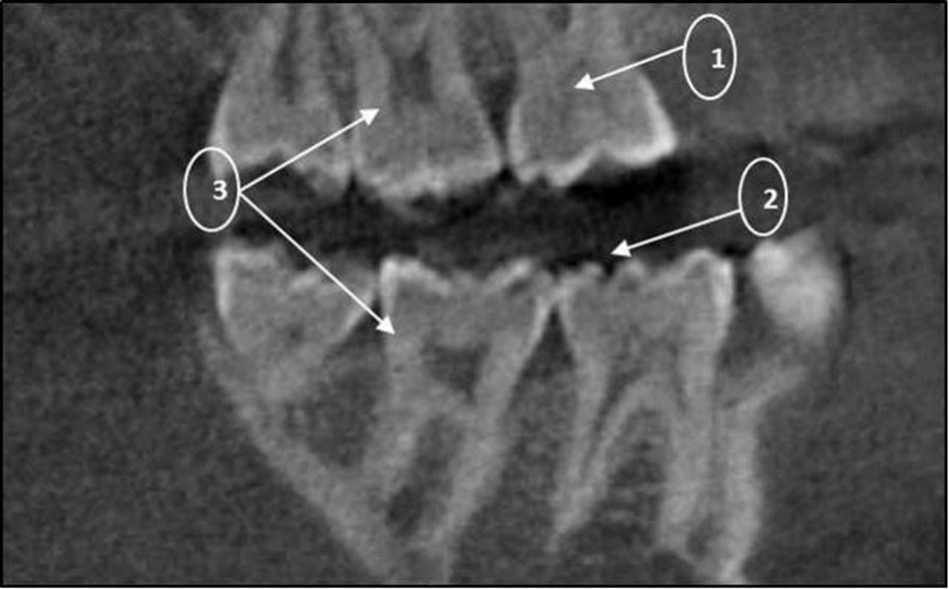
Cropped CBCT image of DBN 4 showing intrapulpal calcifications (1) as well as an unusual occlusal anatomy (2). Features of hypotaurodontism are evident in the 17 and 27 (3)
